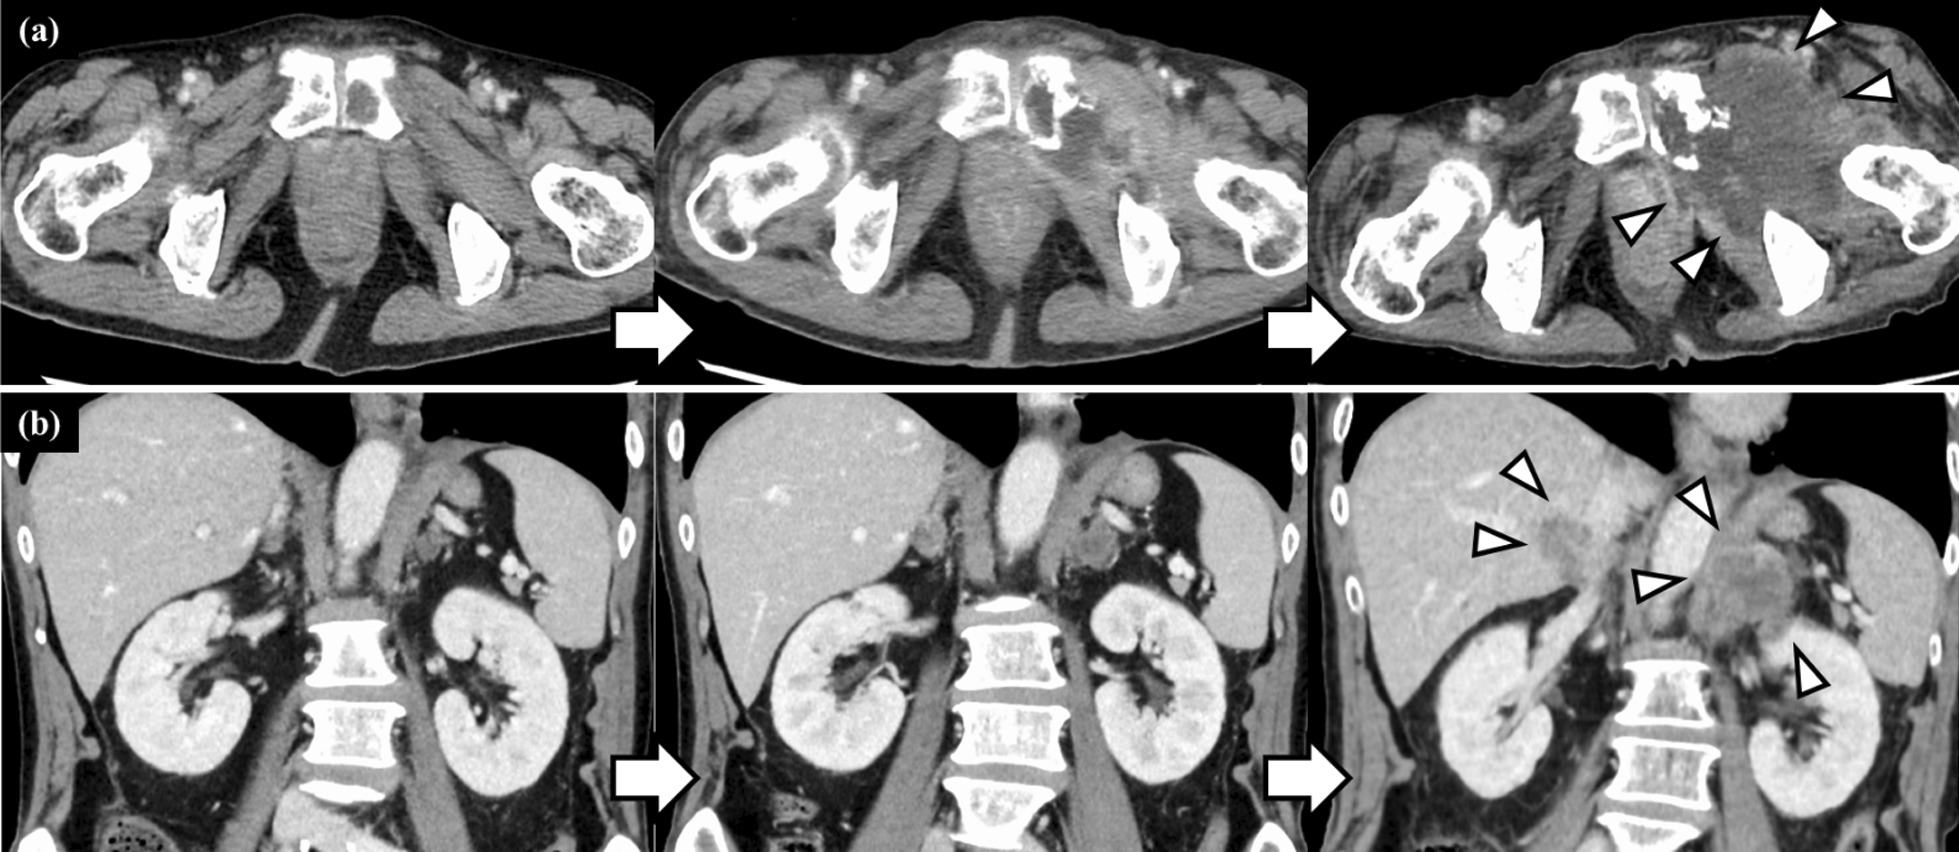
Clinical course of CT findings in distant metastases. a shows the clinical course of the CT findings in patient 1, whose primary tumor is shown in Fig. 2d. The left pubic bone metastasis with an internal low-density area infiltrated the surrounding muscles. b shows the clinical course of the CT findings in patient 2, whose primary tumor is shown in Fig. 2i. Bilateral adrenal metastases with an internal low-density area gradually infiltrated the liver, left kidney, and diaphragmatic crura
